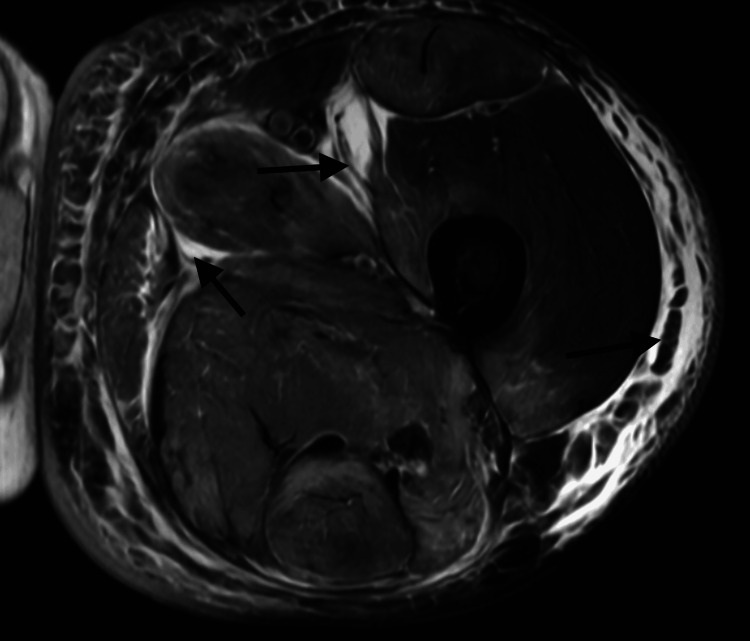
Axial T2-Weighted MRI of the Left Thigh The MRI shows a diffuse hyperintense signal throughout the thigh musculature (black arrows), consistent with extensive muscular edema. There is also subcutaneous and interfascial edema, without evidence of myonecrosis or abscess formation. These findings are characteristic of severe myositis or rhabdomyolysis.
